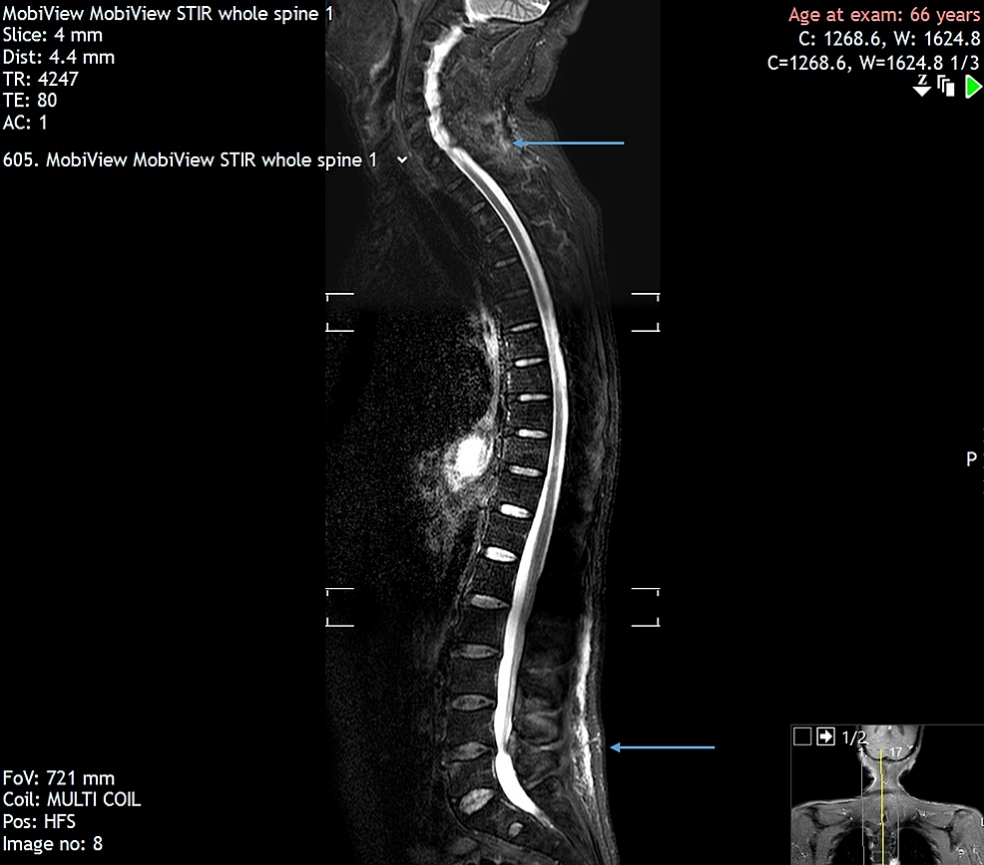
MRI showing facet joint spondylitis at L4-5 and inflammatory changes around the C6 spinous process
